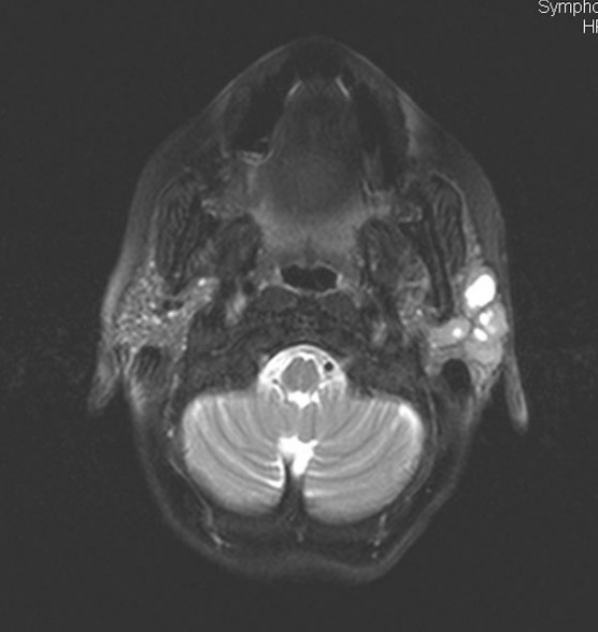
Axial T2-weighted image reveals an enlargement of the left parotid gland and a total replacement of the normal parenchyma with mixed-type solid and cystic lesions.
